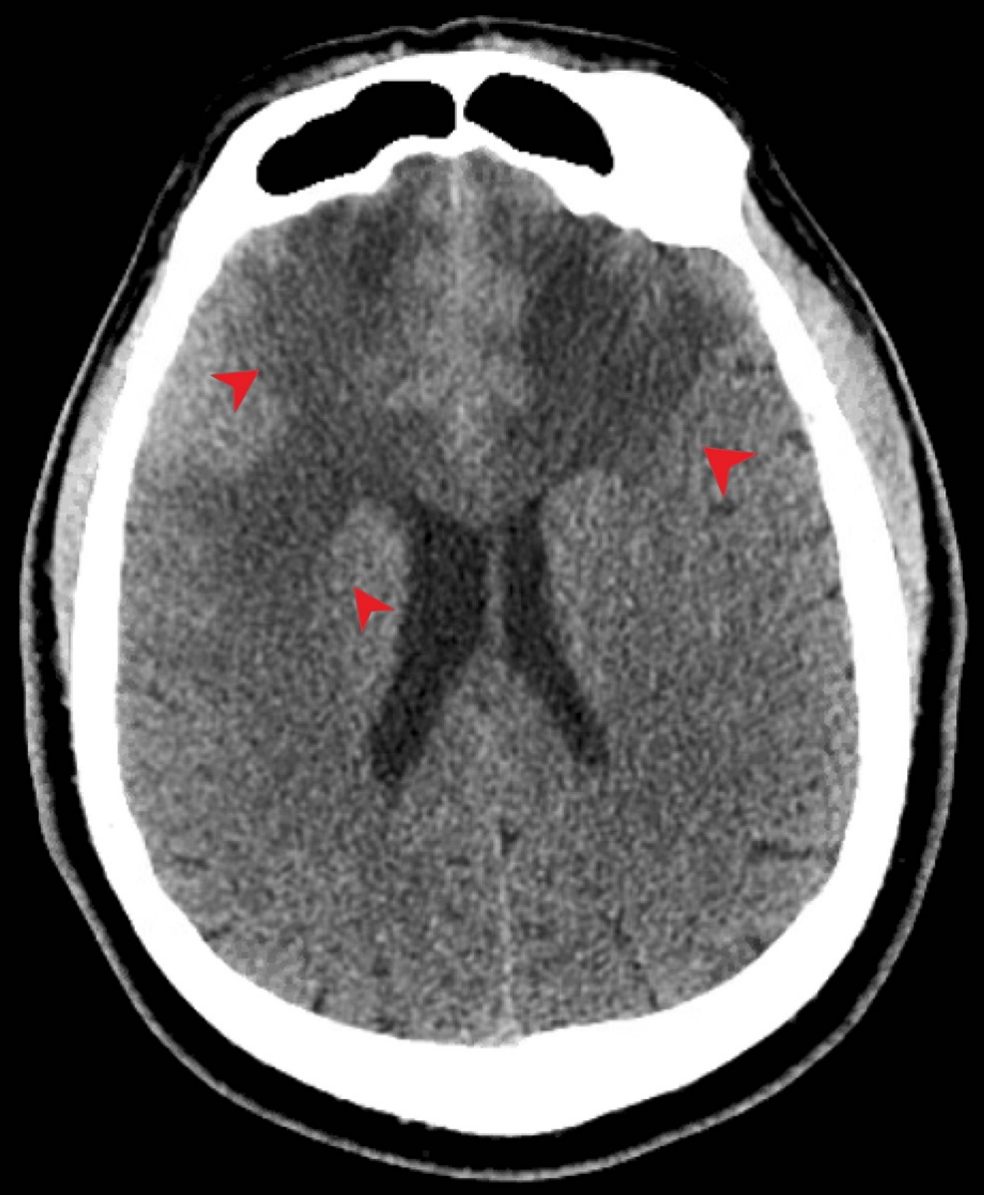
CT scan of the brain (axial view), on arrival, demonstrating large areas of white matter hypodensities in the bifrontal lobes as well as small areas of cortical hypodensities in the right anteroinferior frontal lobe (arrows)
